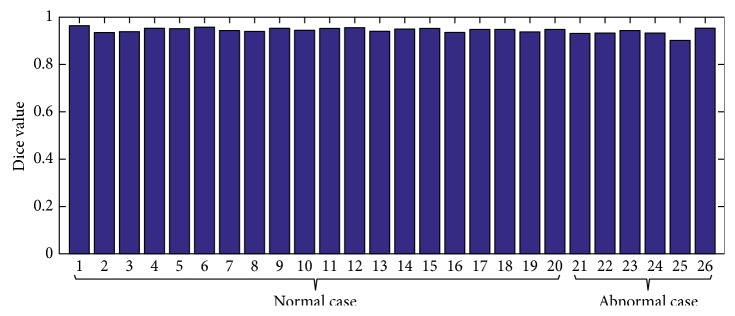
Our technique performed on 26 CT scans with Dice measurement. The first 20 data points are normal cases, and the remaining 6 data points are pathological cases.
